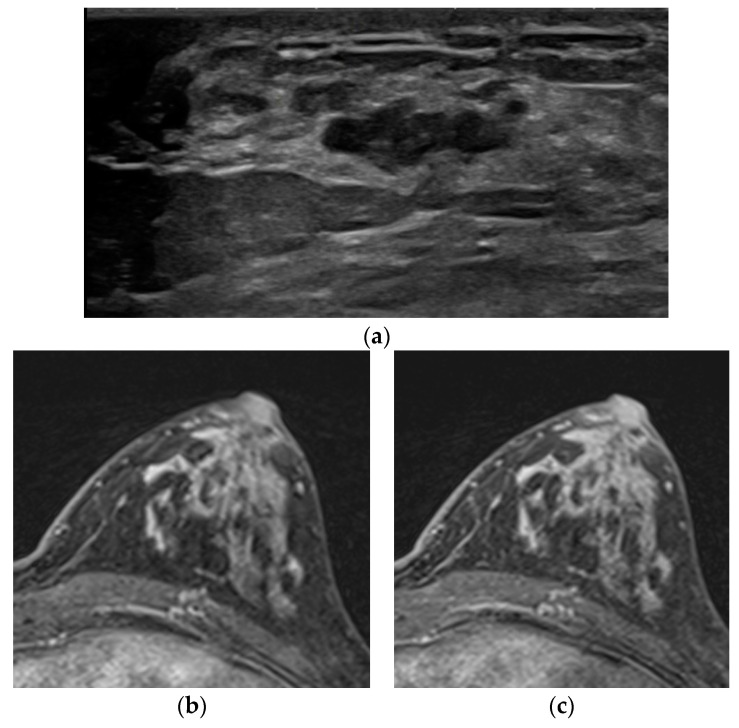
A 53-year-old woman with screening. (a) In the ultrasound, a 2 cm irregular hypoechoic mass was detected in the left breast in the 3-o’ clock direction and confirmed as invasive ductal carcinoma with ductal carcinoma in situ. The final pathology revealed 0.5 cm-sized invasive cancer with 2.4 cm-sized DCIS. No discernible lesion was identified in the subtraction image from the early phase (b) or the delayed phase of T1WI due to the marked level of BPE (c).
